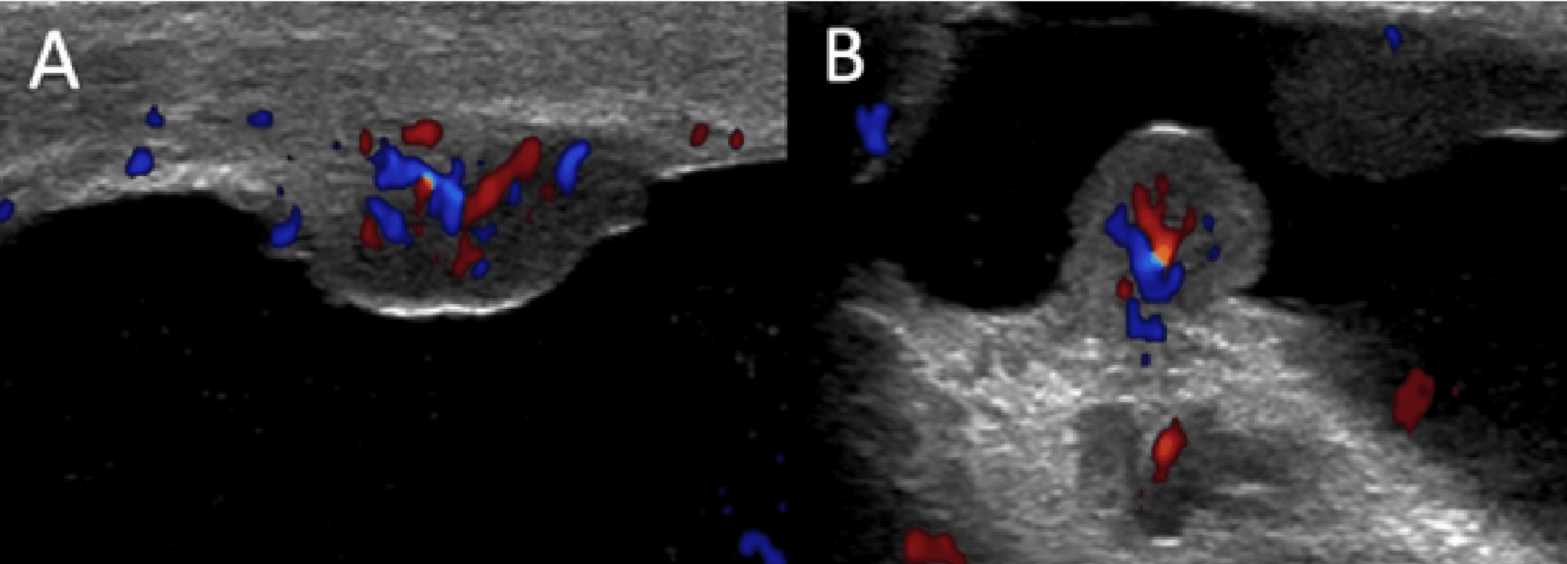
Doppler ultrasound of the right scrotal wall (A,B) Moderately increased vascularity of the soft-tissue nodules within the right scrotum.
